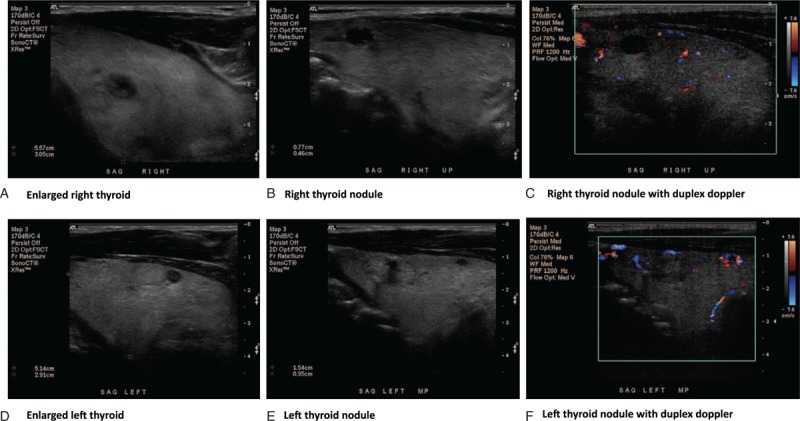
Diffuse enlarged thyroid gland with multiple nodules was shown in thyroid sonogram.
